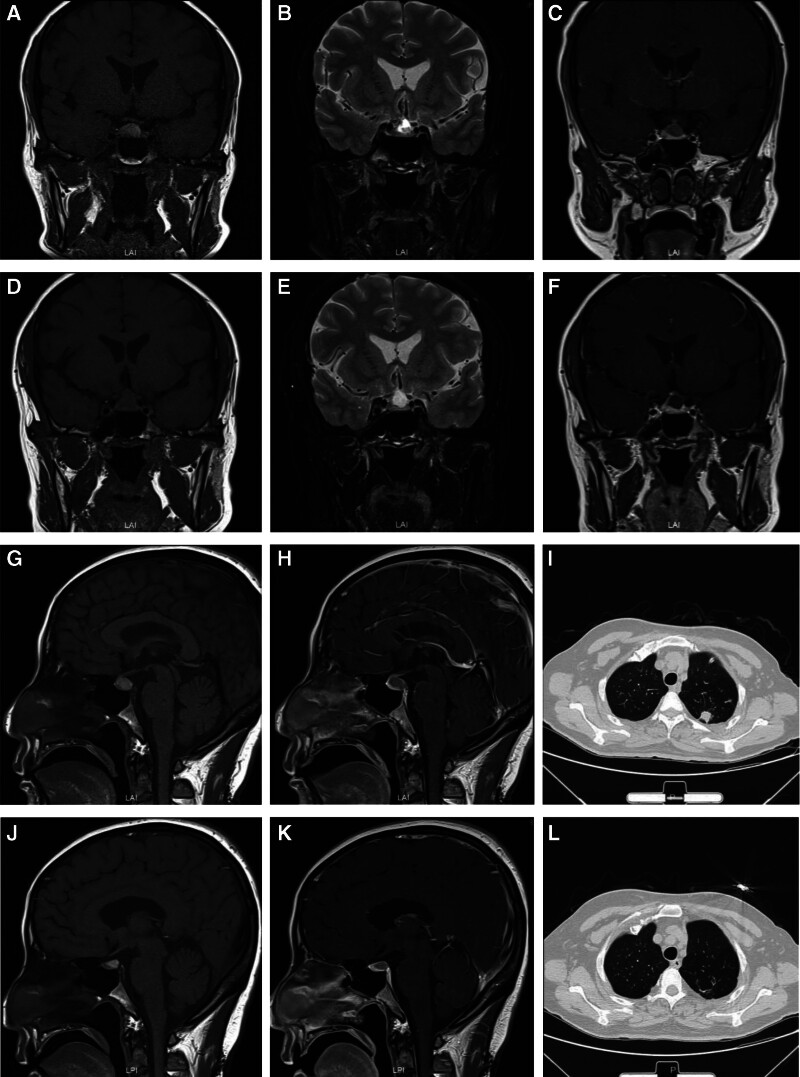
Imaging findings in case 1. Pituitary MRI T1-weighted (A, G): shows high signal intensity in the sellar region, suggestive of pituitary enlargement (12 mm × 15 mm), with Rathke’s cleft cyst (10 mm × 11 mm), no obvious compression of the optic chiasm; T2-weighted (B): shows high signal intensity of Rathke’s cleft cyst; enhanced pituitary MRI (C, H): no significant enhancement observed in the pituitary gland; chest CT scan (I): reveals a subpleural nodule in the left upper lung, possibly representing a chronic inflammatory nodule; follow-up pituitary MRI (D–F, J, K): the pituitary size (11 mm × 15 mm) appears the same compared to previous scans, Rathke’s cleft cyst appears the same size (9 mm × 12 mm) as compared to previous scans; follow-up chest CT scan (L): demonstrates resolution of the subpleural nodule. MRI = magnetic resonance imaging, CT = computerized tomography.
